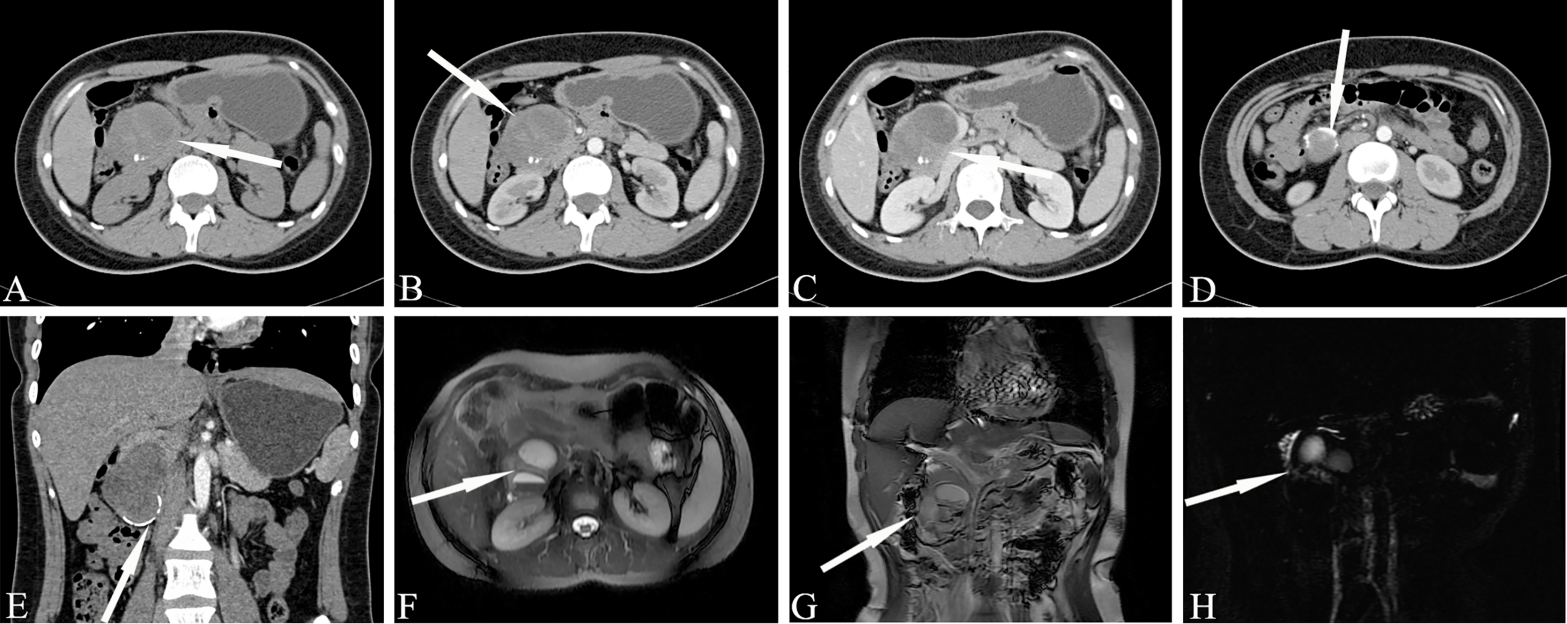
Solid pseudopapillary neoplasm, SPN (Female, 28y, physical examination revealed a pancreatic mass for a week). (A–C) The CT plain scan, arterial phase and venous phase at the same level; (D) Coronal image in arterial phase. (E) Arterial phase cross-sectional image at another level of the same patient. (F). T_2_-weighted cross-sectional image; (G) T_2_-weighted coronal image; (H). MRCP reconstruction map; (A) The CT plain scan showed a low-density mass in the head of the pancreas with cystic degeneration. Calcification was visible in the mass. (B–E) The solid component reinforcement was not obvious. The incomplete arc-shaped calcification of the envelope can be seen. (F–H) MRI shows old hemorrhagic signal with fluid-fluid level. There were no signs of pancreatic duct dilatation. The white arrows in the Figures only indicate the location of the neoplasm.
